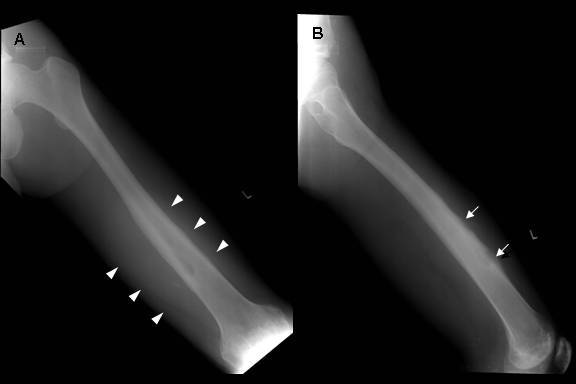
Radiographs of the left femur after RFA demonstrated periosteal reactions (white arrows) (B) with adjacent soft tissue swelling (arrow heads) (A) over the site of previous RFA.
